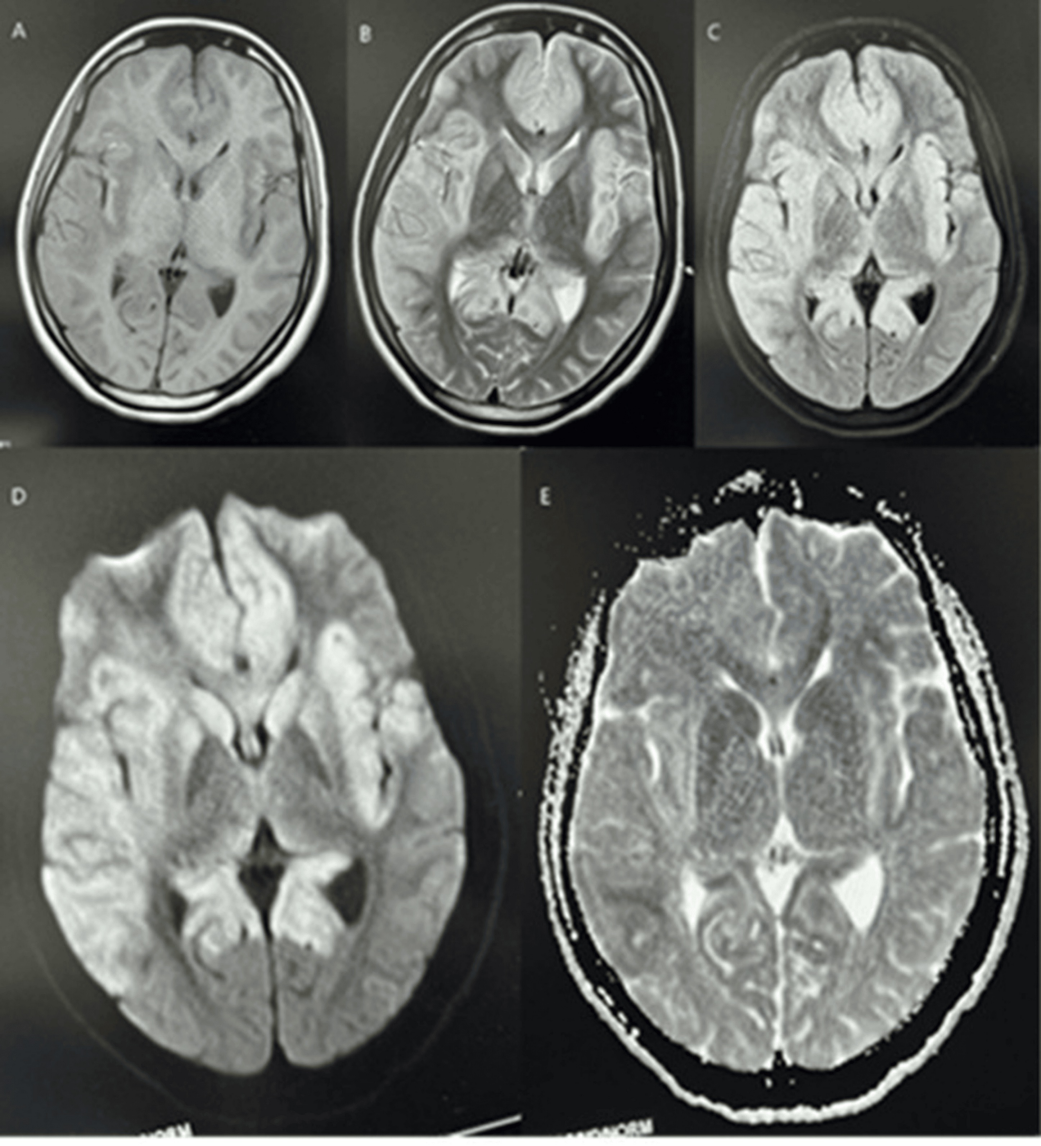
Altered signal intensity on various MRI sequences A. Axial T1-weighted (T1W) sequence shows subtle enhancement in the bilateral frontotemporal lobes, involving the basifrontal regions, perisylvian cortices, and the right temporal lobe, with relative sparing of the medial temporal lobes. B. Axial T2-weighted (T2W) sequence demonstrates multifocal hyperintense patches in the bilateral frontotemporal lobes, including the basifrontal regions, perisylvian cortices, and nearly diffuse involvement of the right temporal lobe, with relative sparing of the medial temporal lobe. Hyperintensities are also noted in deep gray matter nuclei, including the bilateral caudate and lentiform nuclei. C. Axial FLAIR sequence reveals hyperintensity in the bilateral frontotemporal lobes, involving the basifrontal regions, perisylvian cortices, and near-diffuse involvement of the right temporal lobe, with relative sparing of the medial temporal lobe. Similar signal changes are seen in the bilateral caudate and lentiform nuclei. D. Axial diffusion-weighted imaging (DWI) sequence shows hyperintensity in the bilateral frontotemporal lobes, predominantly in the basifrontal regions and perisylvian cortices, with near-diffuse involvement of the right temporal lobe and relative sparing of the medial temporal lobe. Hyperintense signals are also observed in the bilateral caudate and lentiform nuclei. E. Apparent diffusion coefficient (ADC) sequence shows corresponding hypointensity in the same regions—bilateral frontotemporal lobes (including basifrontal regions and perisylvian cortices), with near-diffuse involvement of the right temporal lobe and relative sparing of the medial temporal lobe—suggestive of restricted diffusion. Deep gray matter nuclei, such as the bilateral caudate and lentiform nuclei, are similarly involved.
